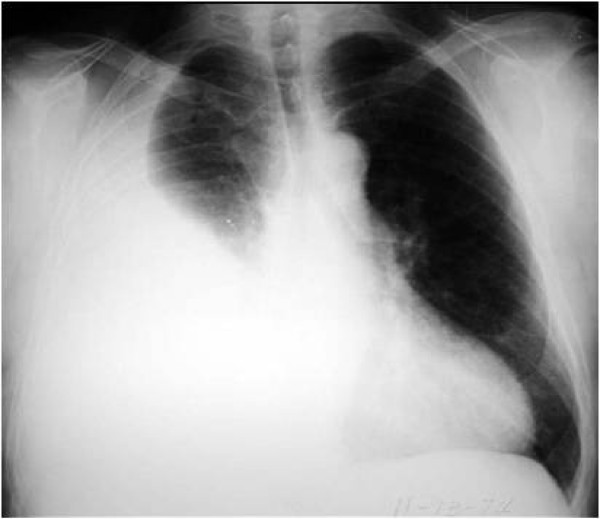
The anteroposterior chest X-ray revealed right pleural effusion.
